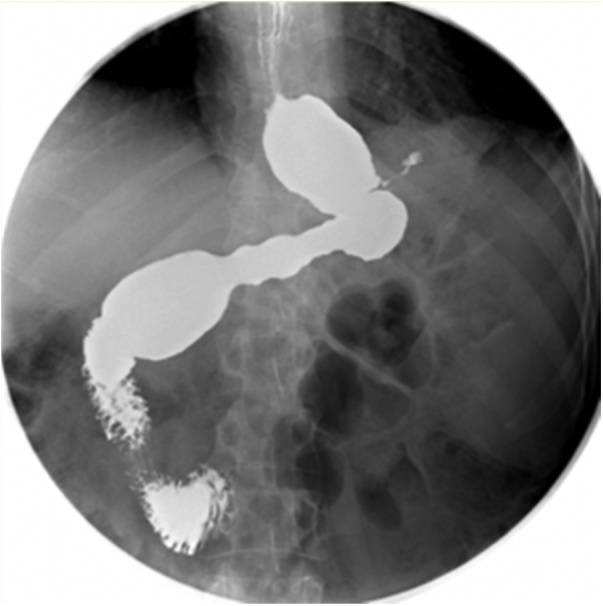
X-ray fluoroscopy barium meal revealing linear contrast leak arising from the left posterior lateral aspect of the proximal gastric pouch, extending to the left sub-diaphragmatic region. No communication with the pleural space at that time.
